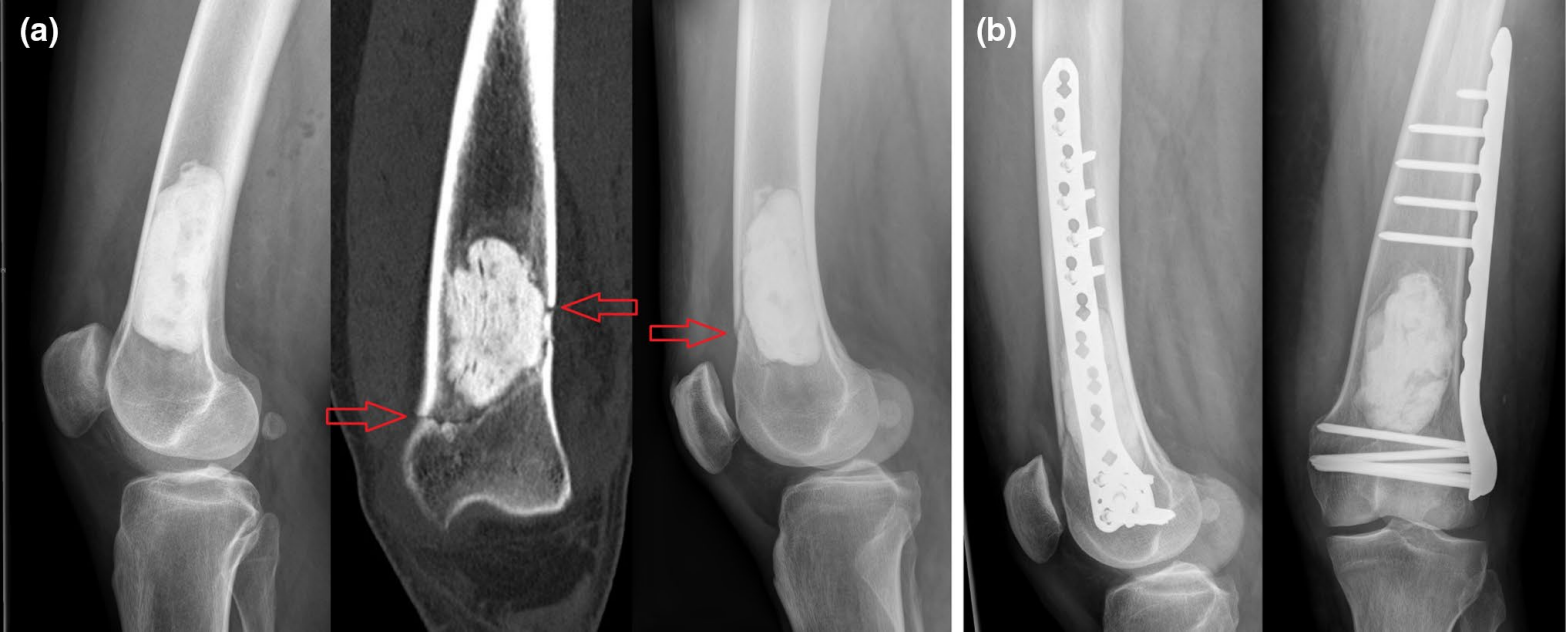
Postoperative X-ray after intralesional excision and filling with polymethylmethacrylate bone cement without additional osteosynthesis (a). Three months after surgery, a fracture was caused by an adequate sport trauma. The non-dislocated fracture is visible in CT and lateral X-ray (red arrows). Revision surgery was performed with osteosynthesis using a large locking compression plate without revision of the bone cement filling (b) (color figure online)
